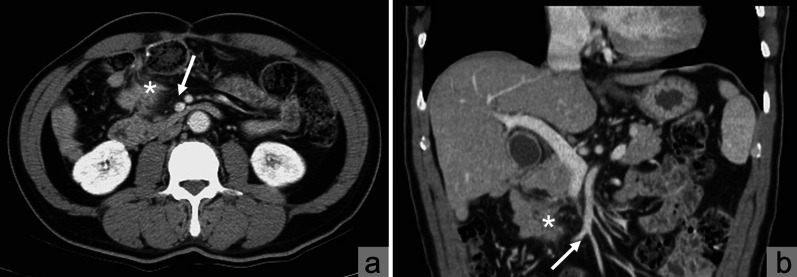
Preoperative contrast-enhanced CT study revealed a the relative position of the right mesenteric root (white arrow) and the lesion (white star sign) on axial view; and b the relative position of the right mesenteric root (white arrow) and the lesion (white star sign) on coronal view
